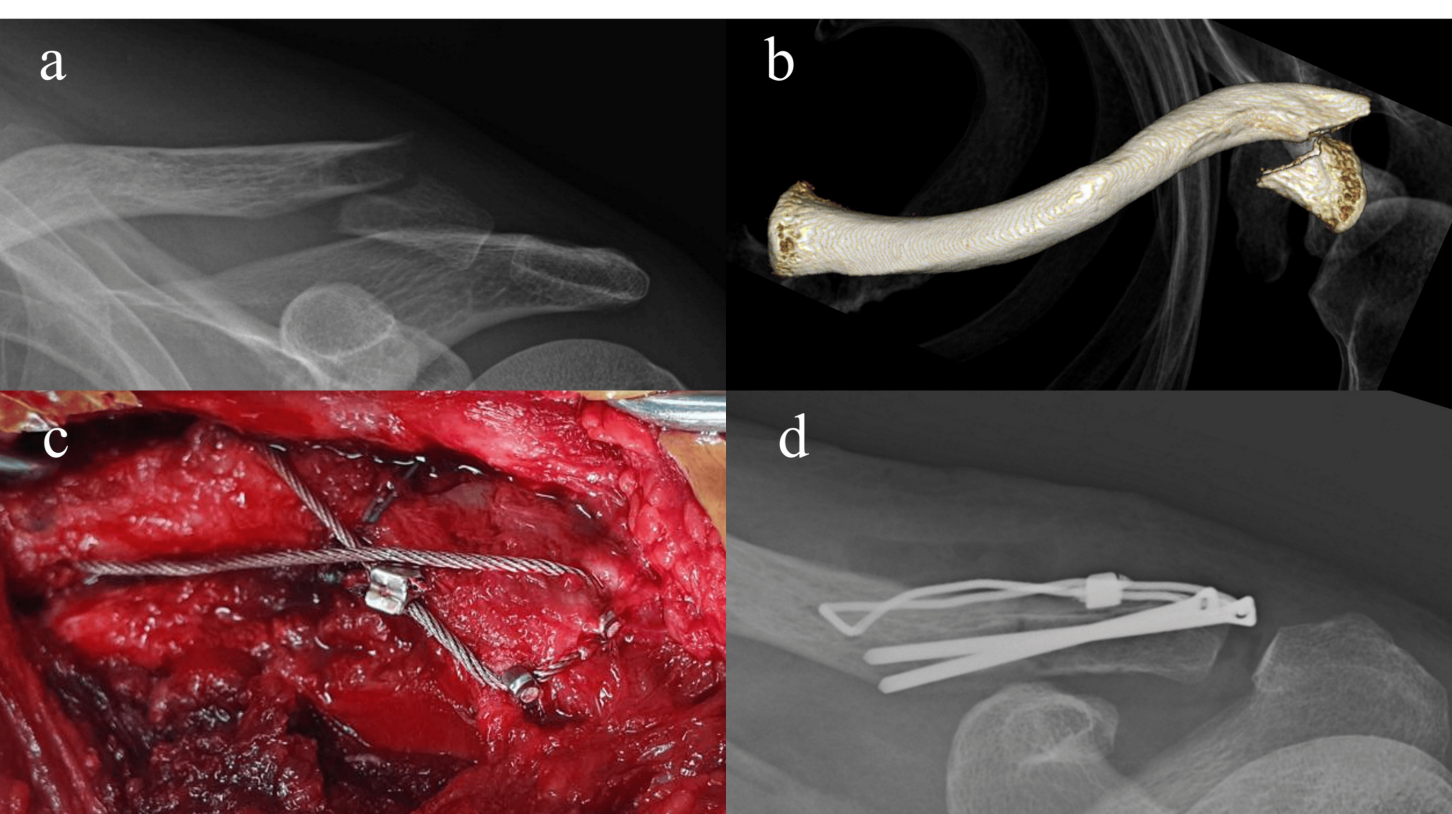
Locked CW for a distal clavicular fracture. (a) Preoperative radiograph showing a distal clavicular fracture. (b) Preoperative three-dimensional computed tomography image showing Craig classification type IIb. (c) Intraoperative image showing locked CW performed, with the pin and sleeve locked. (d) Postoperative radiograph showing satisfactory reduction. CW, cerclage wiring.
